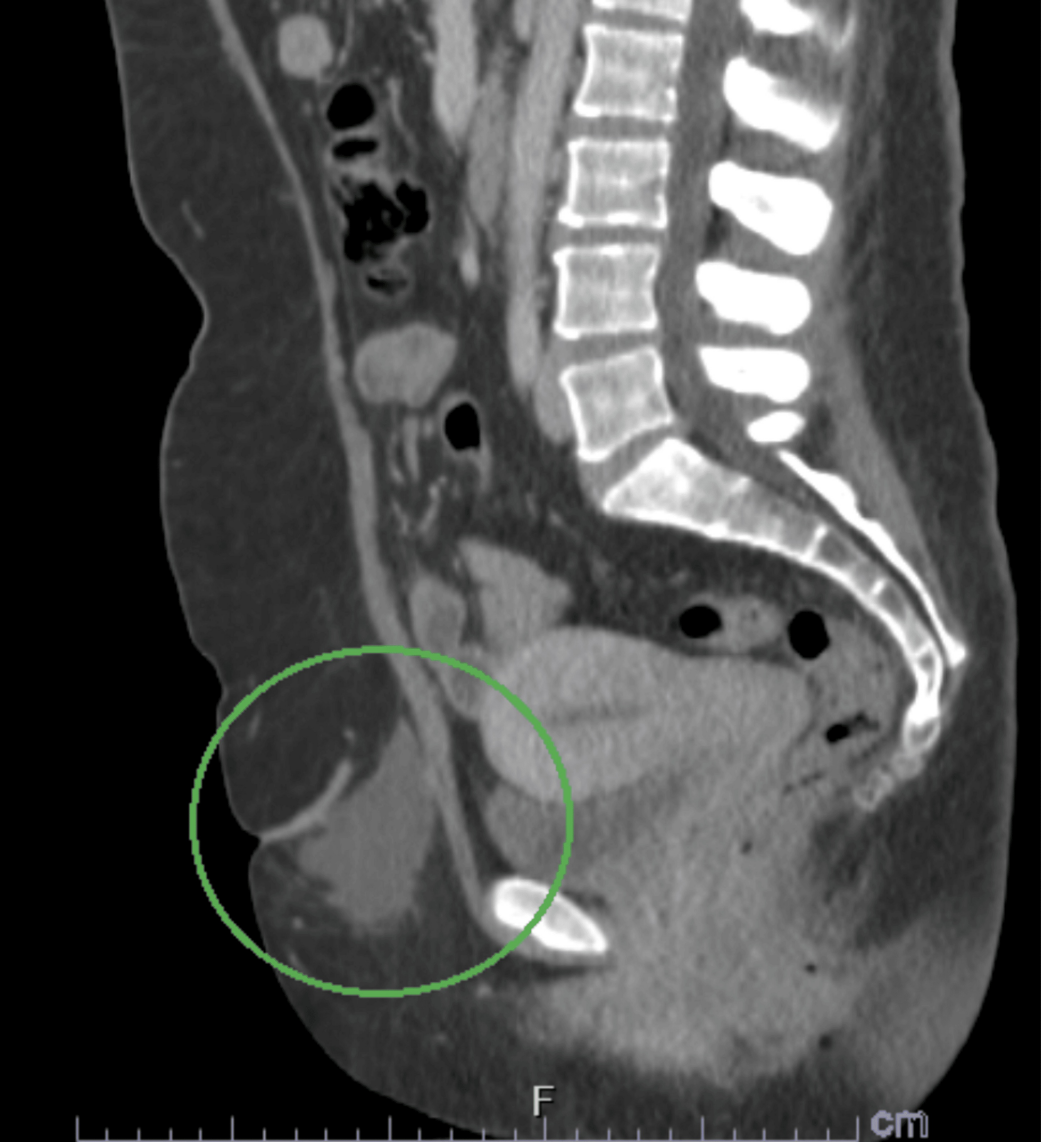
Sagittal CT abdomen view of the enlarging cystic mass that is now 6.7cm x 3.6cm. The nodule has irregular margins with mild spiculations and is directly adherent to the fibrotic, Pfannenstiel scar tissue.
